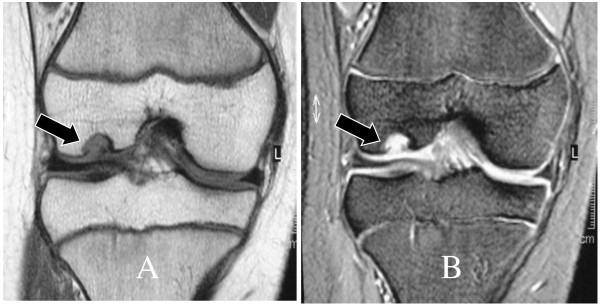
(A) T1-weighted coronal MR image (B) T2-weighted coronal MR image. A cystic structure is seen in the lateral femoral condyle (black arrow). A lesion of low signal intensity on T1-weighted image and high signal intensity on T2-weighted image is evident
